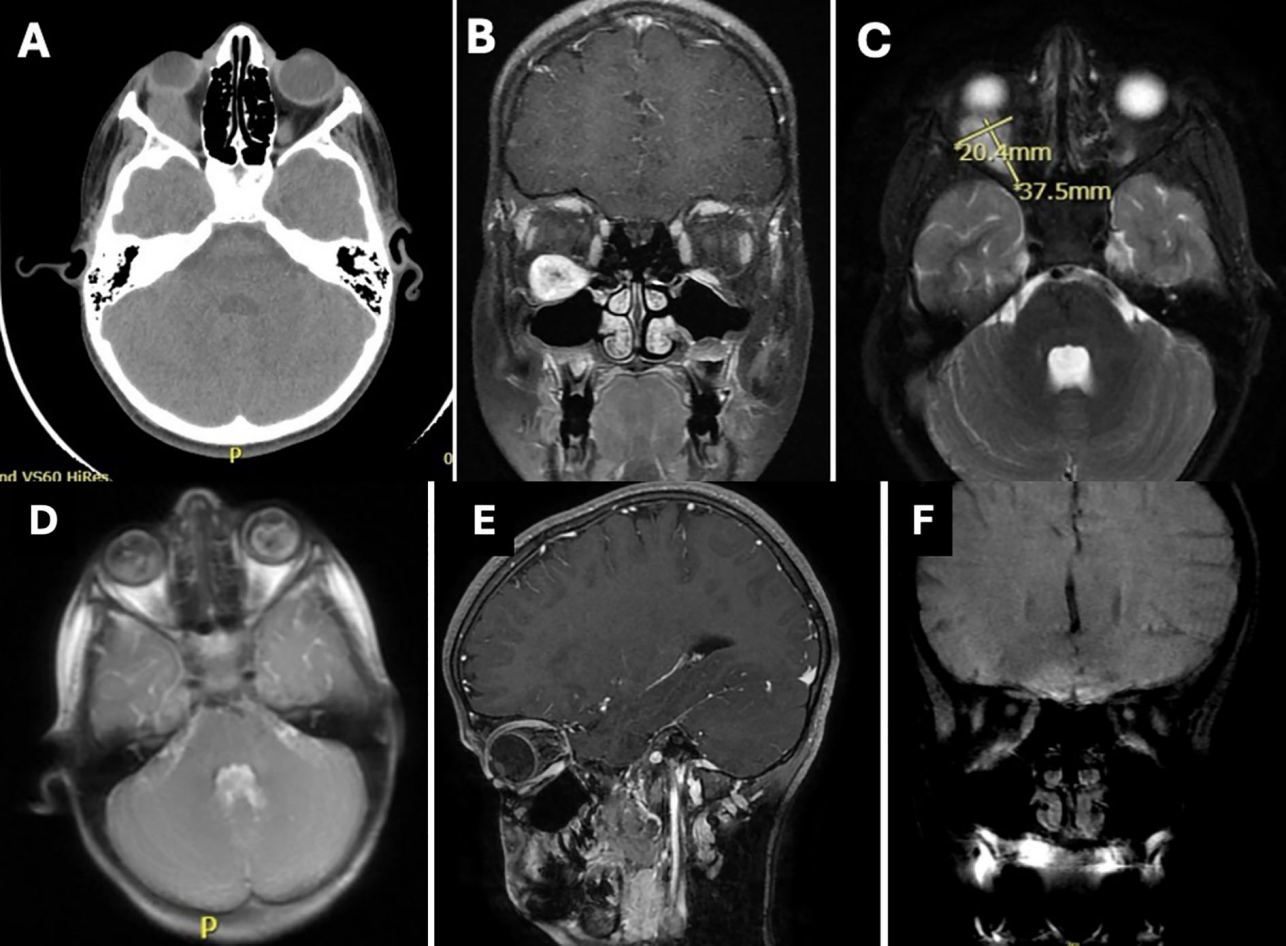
Imaging at presentation demonstrating location and size of the lesion. (A) Axial section CT brain and orbit. (B) Coronal section MRI upon presentation showing mass along the inferior rectus muscle. (C) T2 MRI axial section with lesion dimensions noted. Imaging following resection of the lesion at different times. (D) Axial section MRI, (E) sagittal section MRI; both after surgery showing complete resection of the lesion. (F) coronal section MRI after two years demonstrating no recurrence and return of the normal orbital anatomy.
